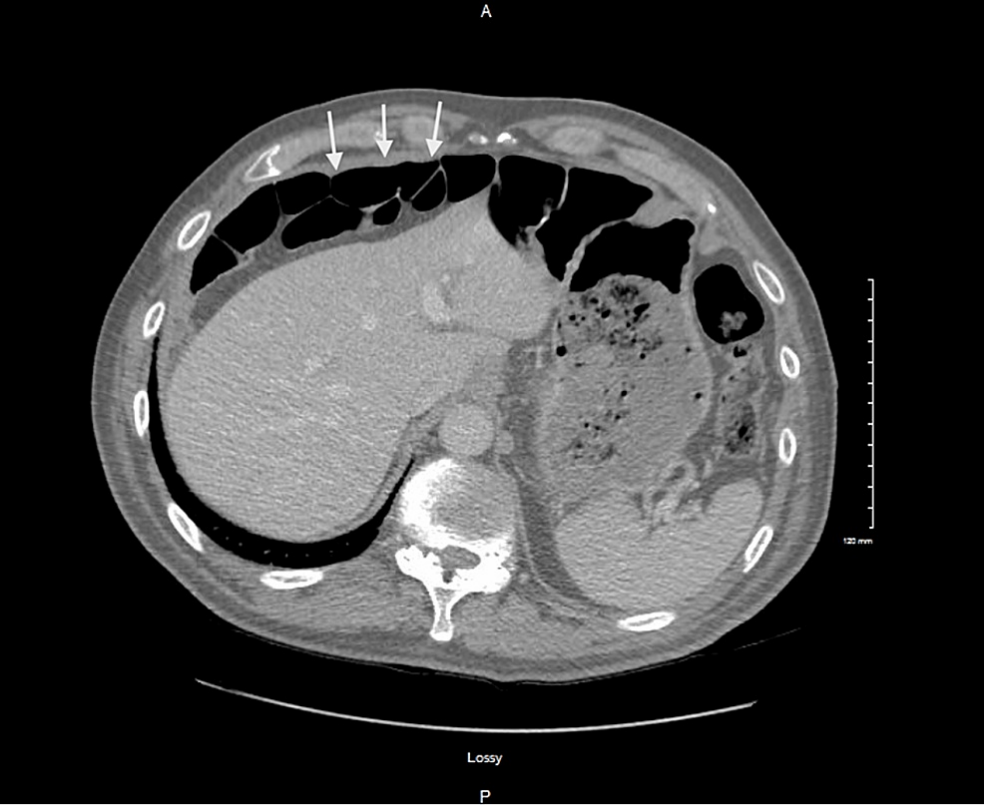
Abdomen/pelvis CT of the abdomen/pelvis with white arrows pointing at the bowel loops interposed between the abdominal wall and the liver, which are dramatically changed from the previous study CT: computed tomography
